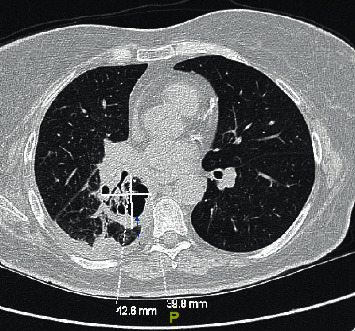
CT chest revealing the right upper lobe cavitary lesion (blue arrow).
